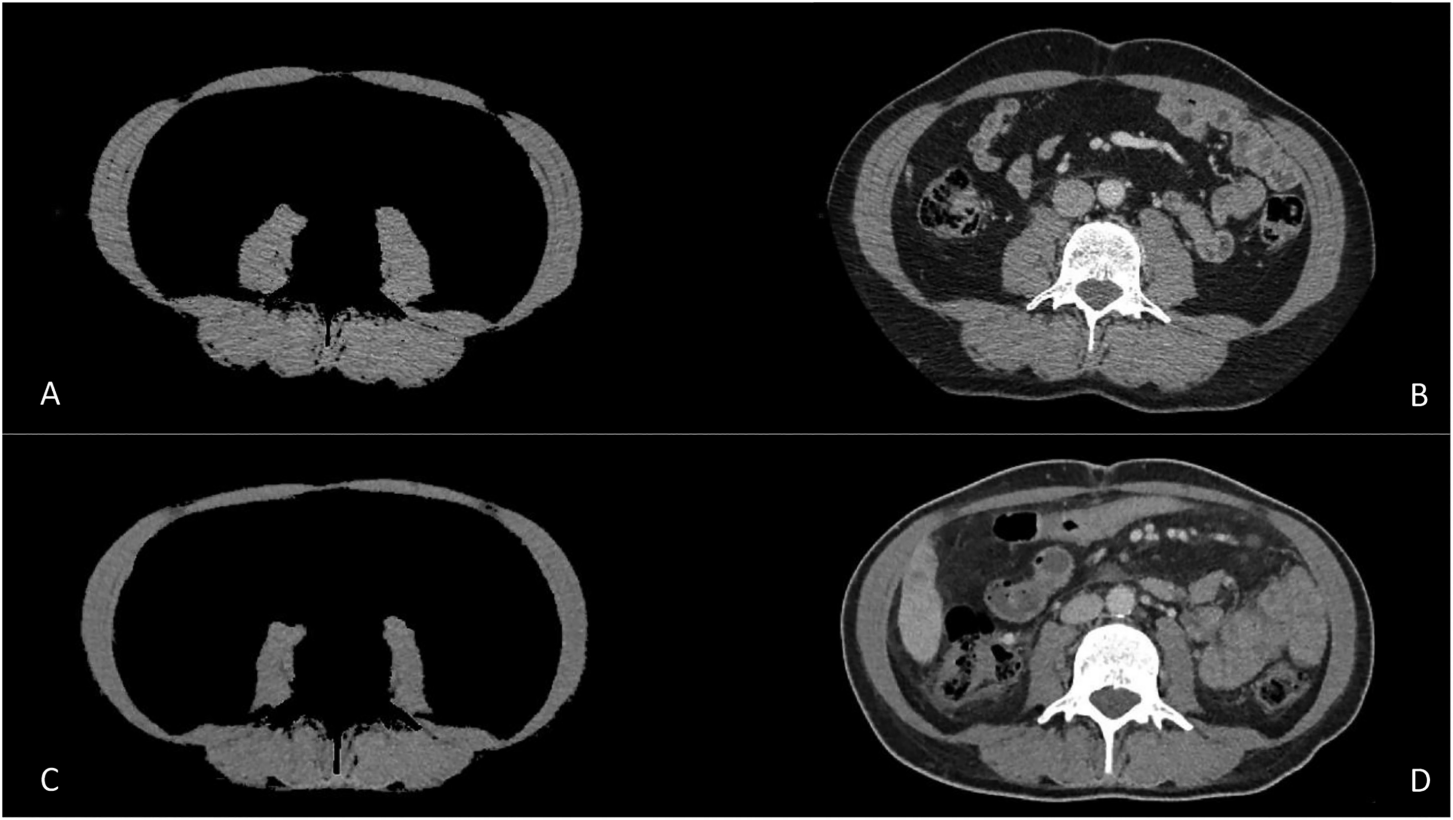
Two lumbar L3 CT scan slices at W0 (A and B) and at W16 (C and D) depict a male patient included in mITT2 population (APA arm), exhibiting a 20.6% decrease in muscle mass between W0 and W16. The patient main characteristics included an ECOG performance status of 0, moderately active GPAC score, a BMI of 24.9 kg/m^2^, the presences of liver metastasis, with SMI of 48.9 cm^2^/m^2^ at W0 and of 37.82 cm^2^/m^2^ at W16 SMI, OS of 4.5 months and TUDD of global health status of 0.82 months.
